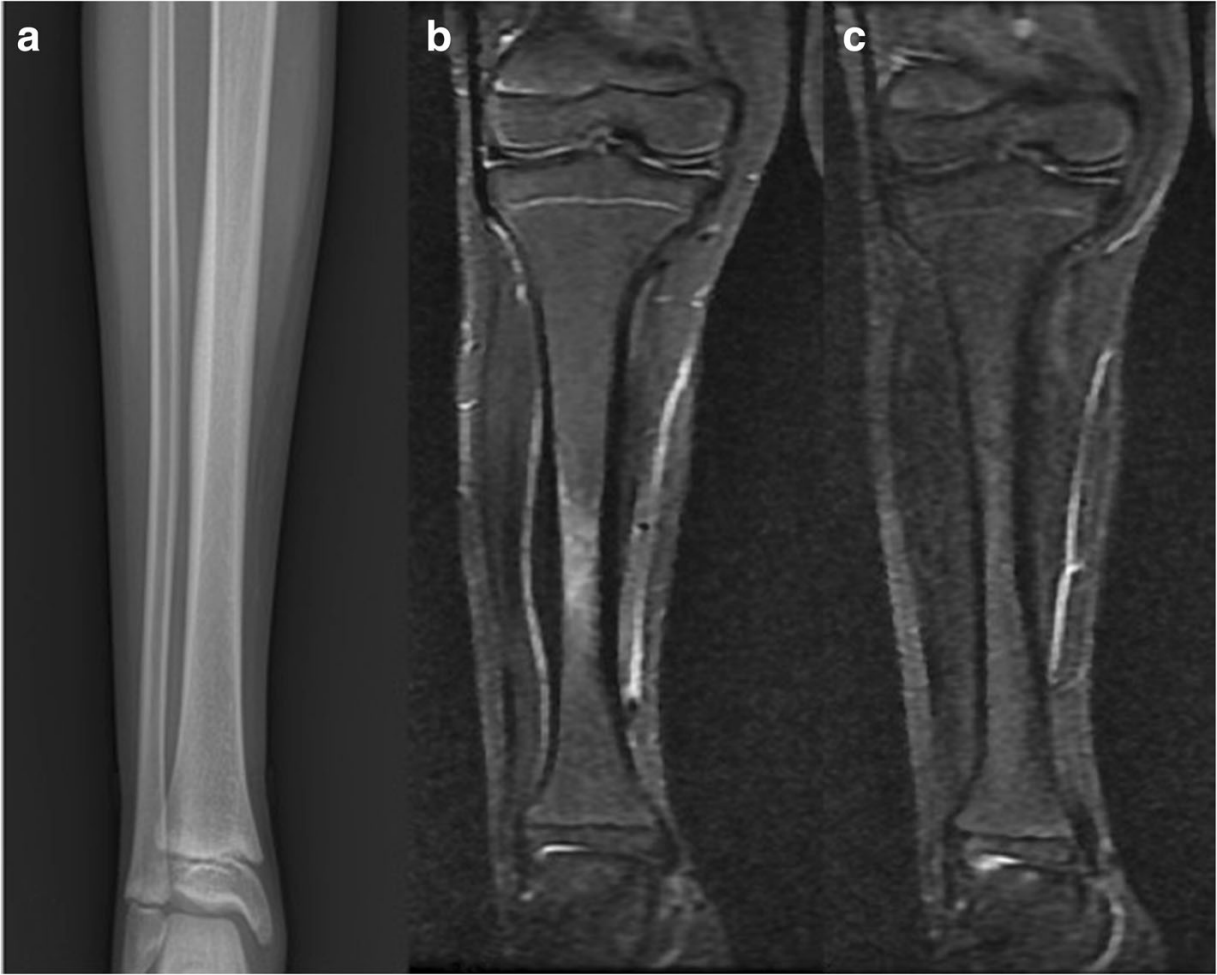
a Conventional radiograph of the 8-year-old patient diagnosed with transient edema in the tibia. b Preoperative coronal T2-weighted MR image. c Postoperative T2-weighted MR image
